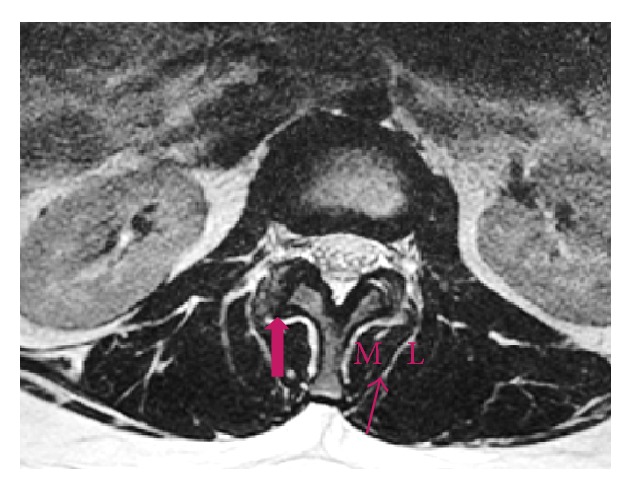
MRI axial image of the lumbar spine with the block arrow pointing to the facet joint, which is the most prominent area after the spinous process and is easily palpable after the lumbar fascia is incised. The thin arrow denotes the plane of dissection between multifidus medially (M) and longissimus laterally (L). This plane is approximately 2 to 2.5 cm lateral to the midline.
